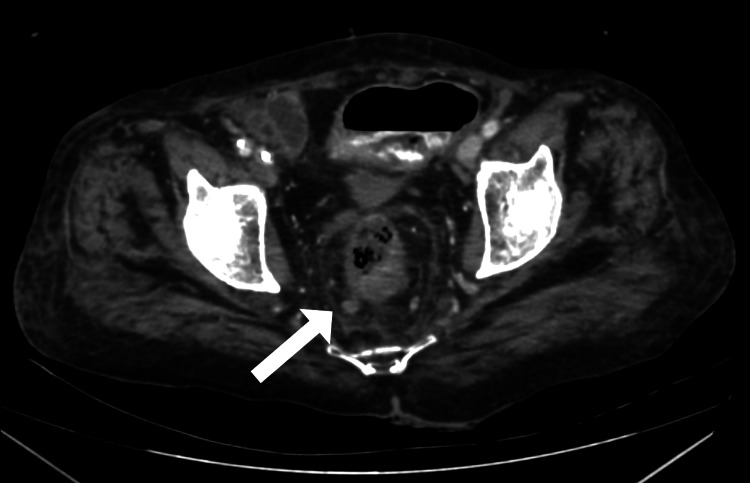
Axial post-contrast CT image of the abdomen showing thickening of the mesorectal fascia, regional fat stranding, and perirectal lymphadenopathy around the mural thickening in the rectosigmoid junction
